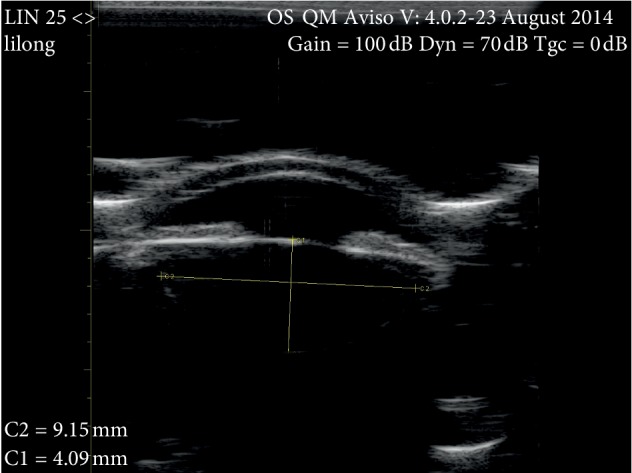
A 25 MHz ultrasonographic image of the left eye of a 40-year-old female. The gain was set to 100 dB, the dynamic range to 70 dB, and the time gain compensation to 0 dB. The entire contour of the lens, including the equator anterior and posterior surfaces, was clearly visible. Using the calipers, yellow lines, shown in the image, the axial lens thickness and equatorial lens diameter were calculated as 4.09 mm (C1) and 9.15 mm (C2), respectively.
